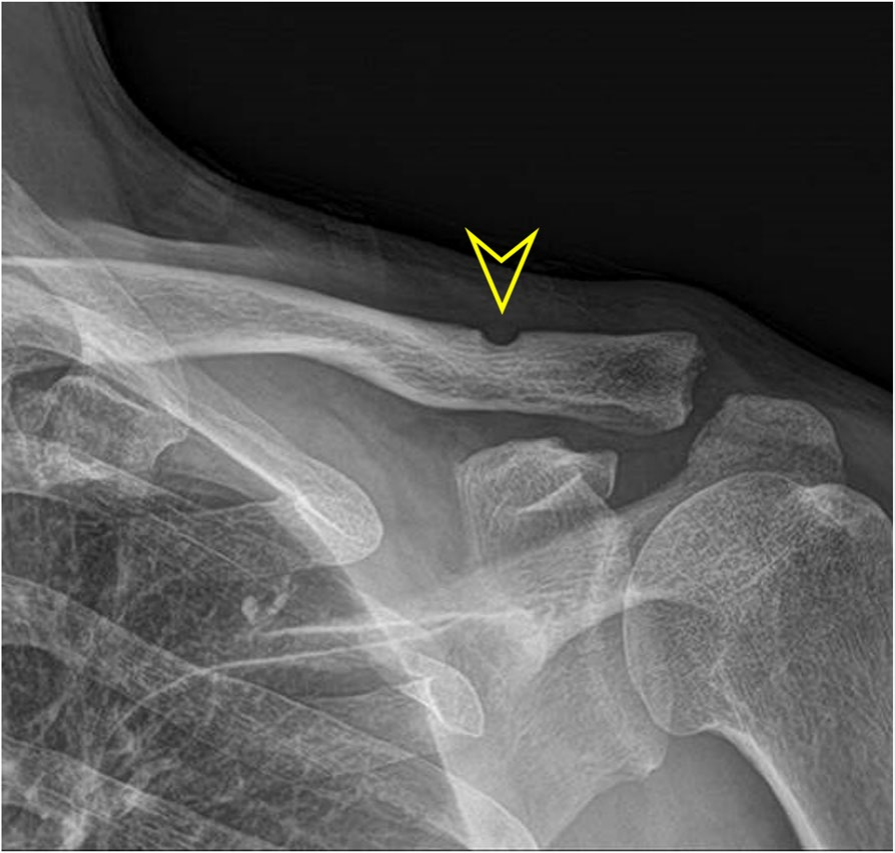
A plain radiograph taken at postoperative 18 months shows erosion of the superior cortex of the clavicle (arrow head). However, the CC and AC distances are well maintained
